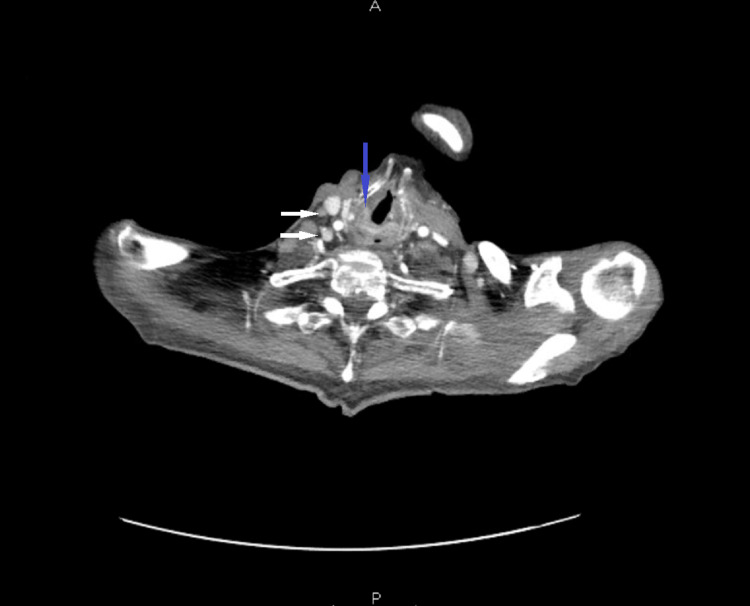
CT scan of the soft tissue of the neck with contrast showing soft tissue fullness in the cervical esophagus (blue arrow) with multiple necrotic lymph nodes throughout the cervical chains (white arrows)
